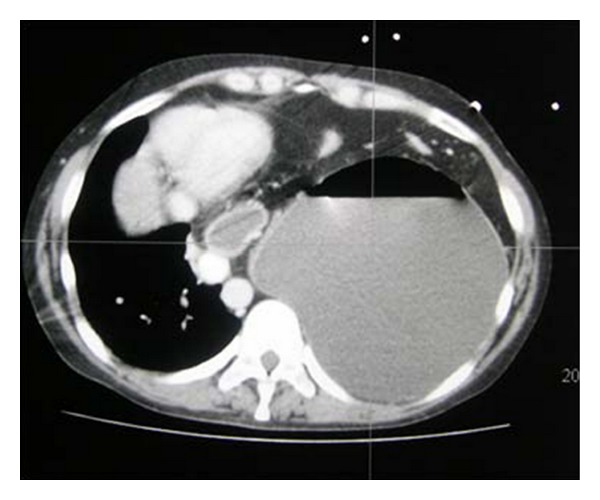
Axial CT on the same patient as Figure 13 showing diaphragmatic rupture and herniation of the stomach into the left hemithorax.
